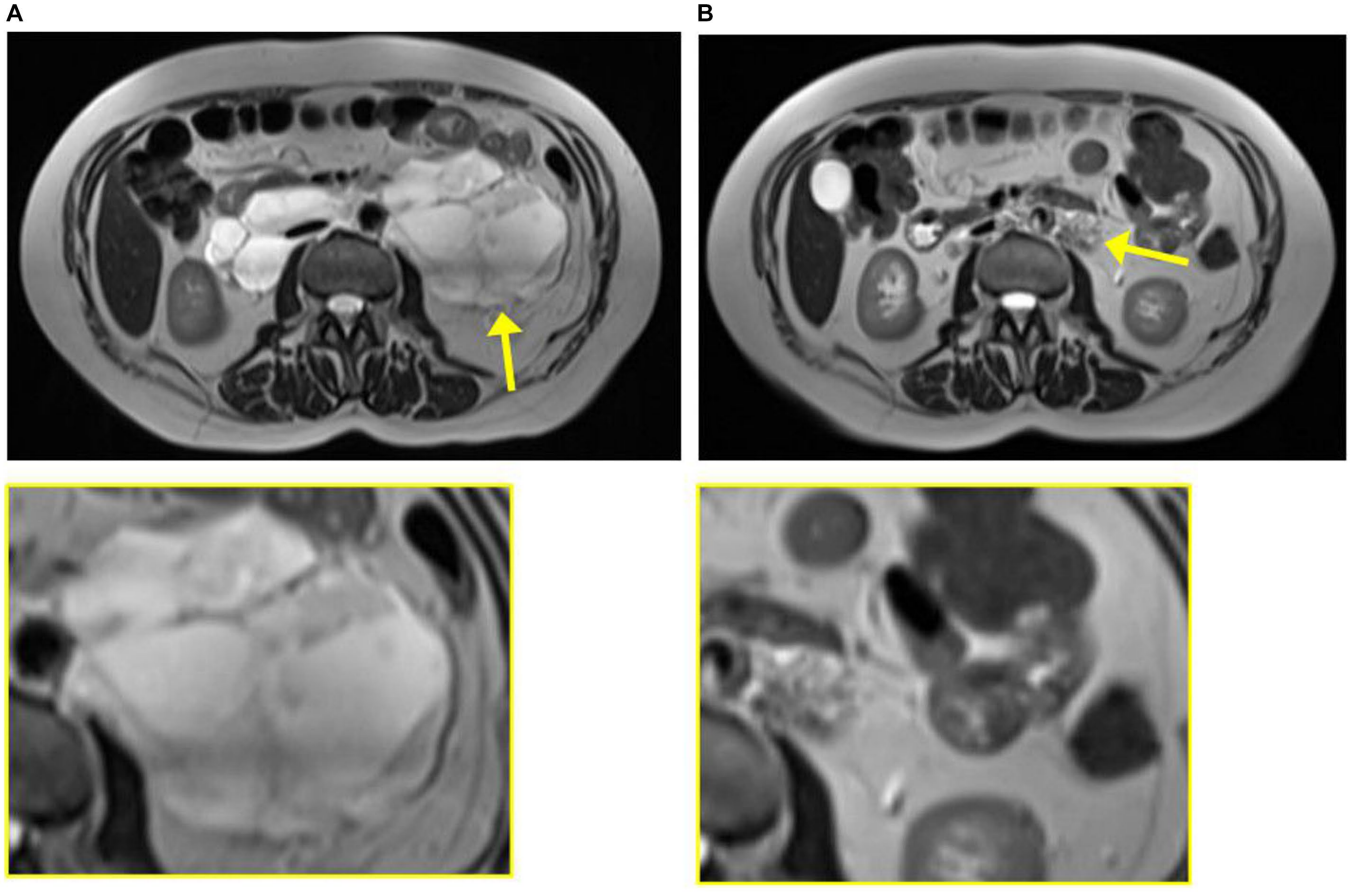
Magnetic resonance imaging from case 1 from 2020 at diagnosis of leiomyoma (A) and 2023 at diagnosis of lymphangioleiomyomatosis (B). The tumor is indicated by the yellow arrow and enlarged in the yellow box.
